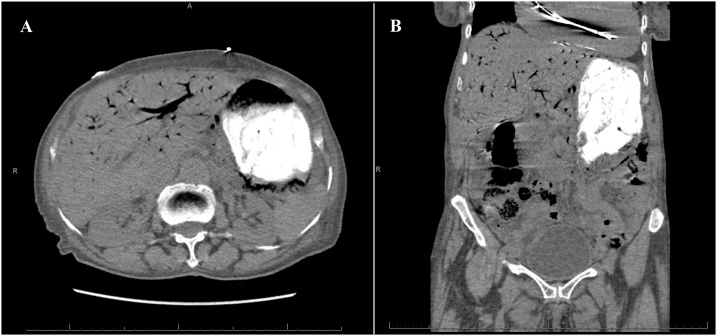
Non-contrast computed tomography scan on presentation revealing gastric pneumotosis and extensive portal venous gas.
